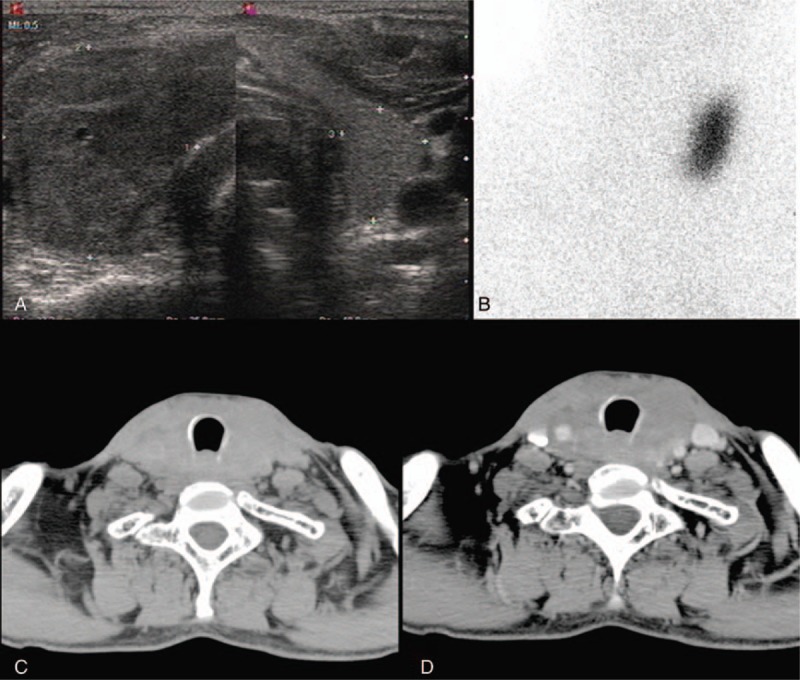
Clinical radiological data. (A) Thyroid ultrasound revealed a hypoechoic mass in the left lobe and heterogeneous echo in the right lobe. (B) 99TcmO-4 thyroid nuclear imaging showed a cold nodule in the right lobe of thyroid. (C) CT revealed a diffused enlargement of thyroid with the homogeneously low intensity in the noncontrasted phase. (D) The slightly homogeneous enhancement of thyroid in contrasted phase on CT. CT = computed tomography.
